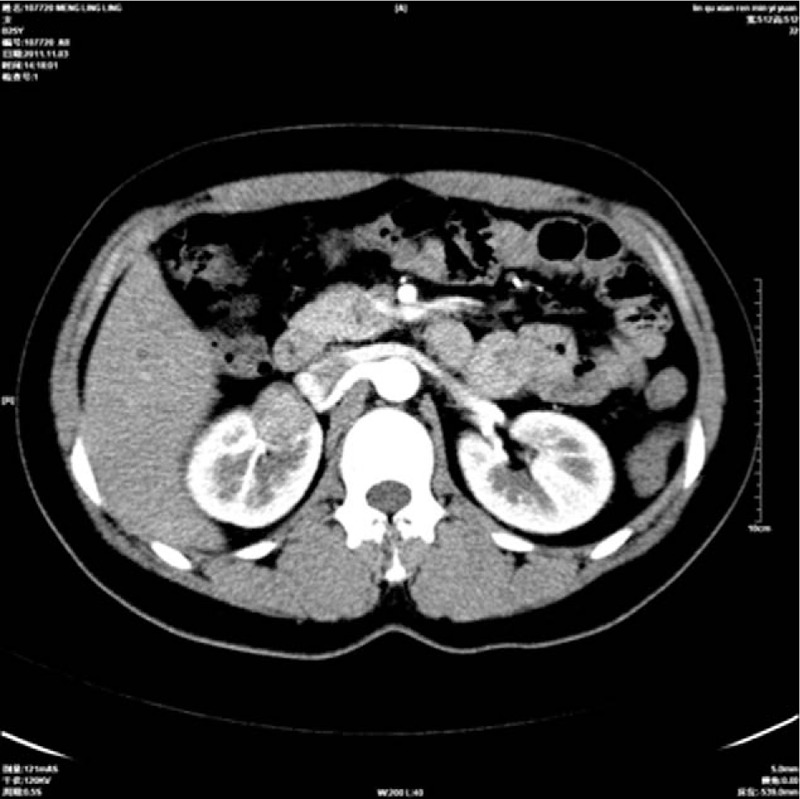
Post-contrast CT revealed the mass was with moderately inhomogeneous enhancement. CT = computer tomography.
